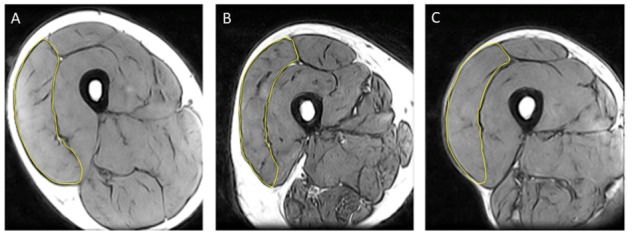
Representative MRI images highlighting vastus lateralis (VL) cross sectional area (CSA) from (A) young control; (B) older control; and (C) old endurance athlete.
